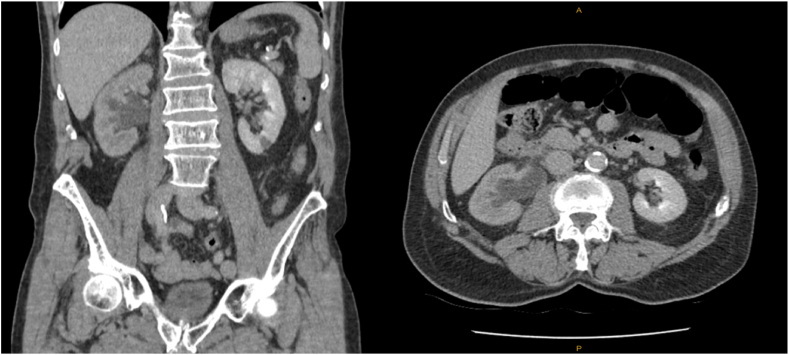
CT Abdomen/Pelvis demonstrating right -sided hydroureteronephrosis.
